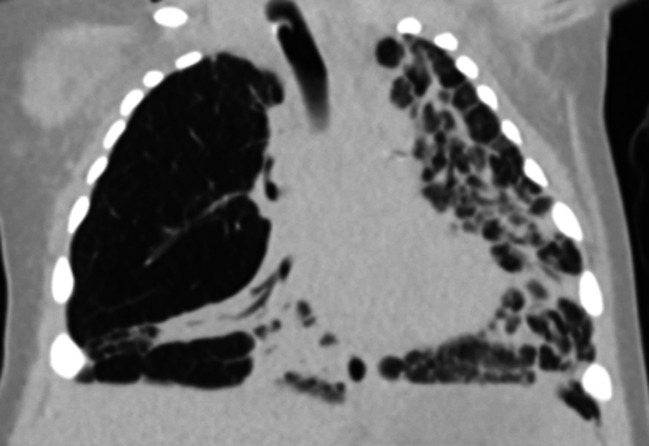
Chest computed tomography of an 8 month old former 26 week premature infant with severe BPD demonstrating dramatic pulmonary heterogeneity with alveolar simplification of the right upper lobe and diffuse fibrosis of the left lung. The patient is also severely hyperinflated with flattened diaphragms.
